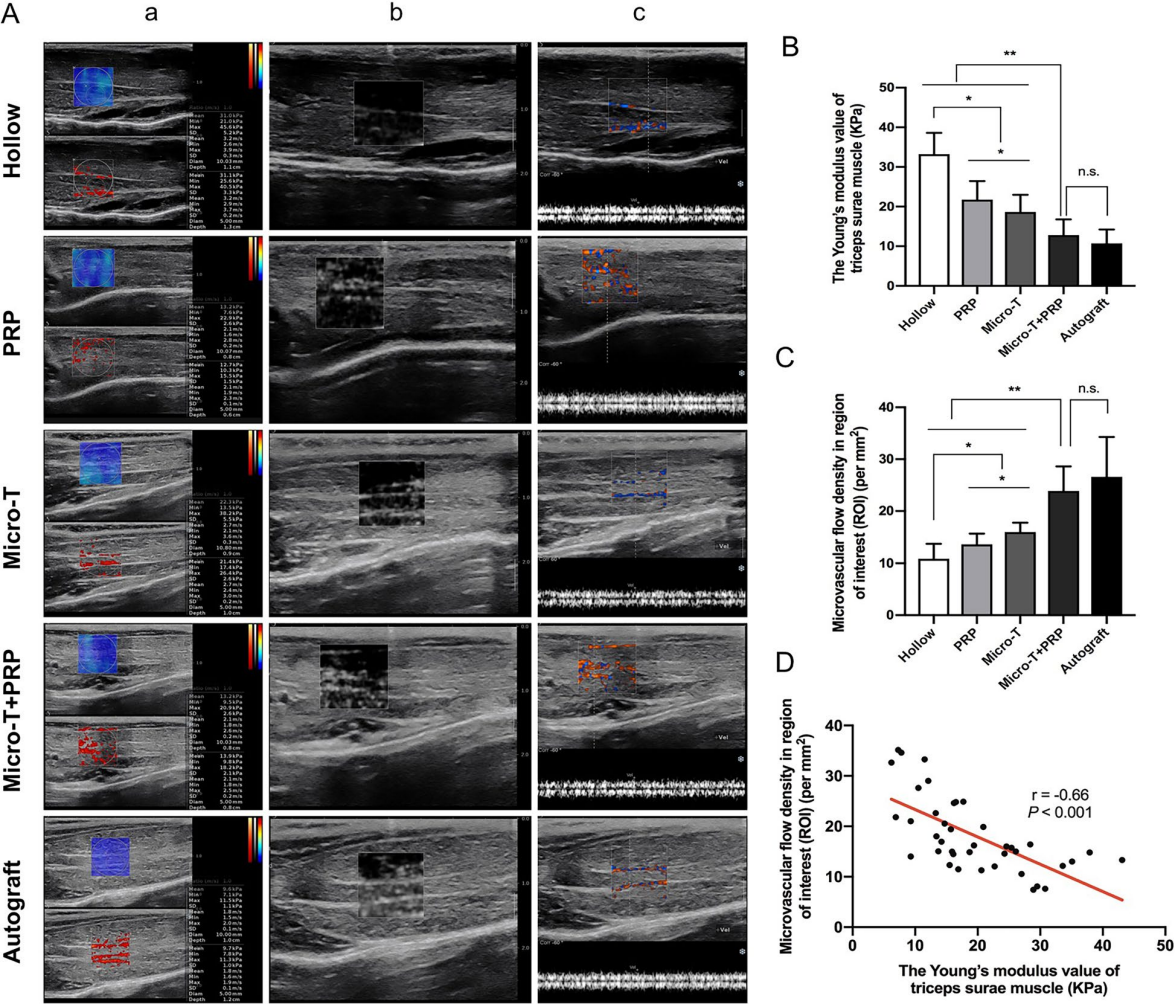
Shear wave elastography (SWE) and Angio PlaneWave UltrasenSitive (AngioPLUS) imaging evaluation. (A) SWE and AngioPLUS images were diaplayed simultaneously on the screen (a), and the monochrome pattern of the microvascular muscle was also displayed (b); (c) Spectral Doppler ultrasonography confirmed that the image shown by Angioplus was microvessel in the triceps surae muscle. Statistical analyses were performed by calculating (B) the Young’s modulus value of triceps surae muscle, and (C) the microvascular flow density in region of interest. ^*^*P* < 0.05, ^^*P* < 0.01, and n.s. not significant. Micro-T microtissues, PRP platelet-rich plasma
